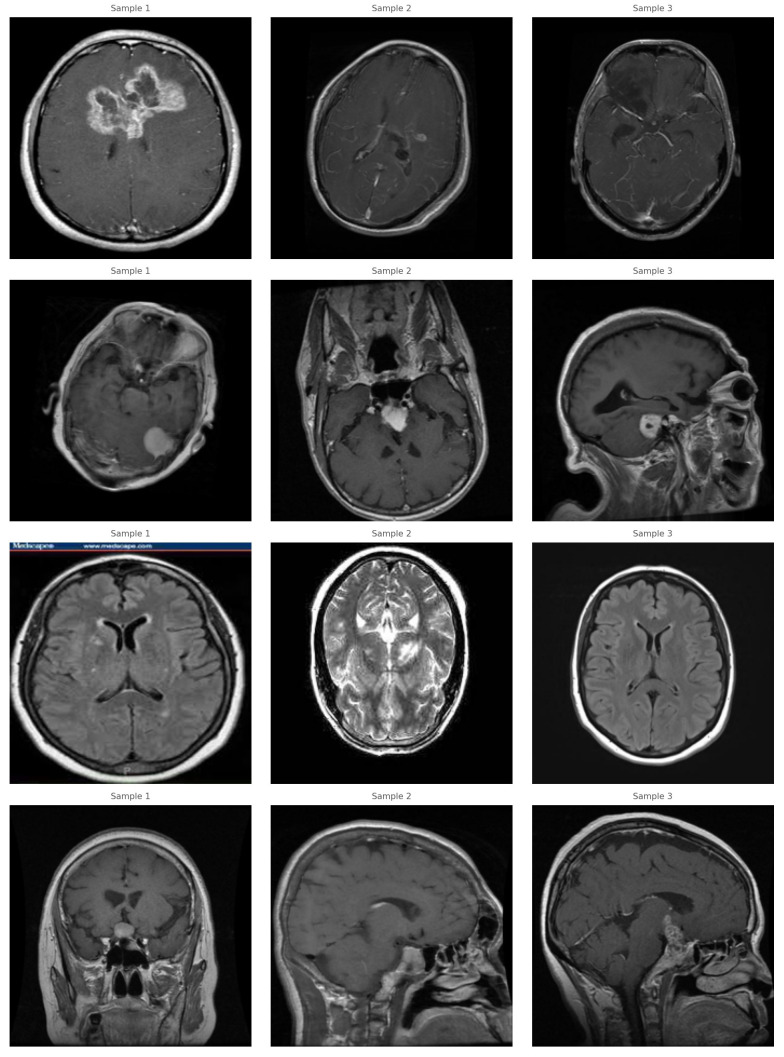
Representative brain MRI samples from the four-class dataset. Each row corresponds to one tumour category (top to bottom: Glioma, Meningioma, No Tumour, Pituitary); each column presents a distinct acquisition plane or scanner protocol. Glioma scans exhibit heterogeneous signal, irregular mass effect, and perilesional oedema. Meningioma samples show a well-circumscribed extra-axial mass. No-tumour images contain normal brain parenchyma. Pituitary adenomas present as sellar or suprasellar lesions. The visual diversity within each class underscores the need for robust, attention-guided feature extraction.
